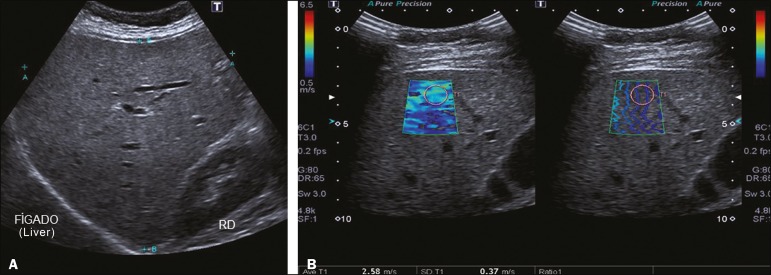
A: Ultrasound examination of a liver with mild steatosis and signs of chronic liver disease, as characterized by blunt edges, irregular contours, and heterogeneous parenchyma. B: A 2D-SWE study showing greater stiffness of the liver parenchyma (2.58 m/s), consistent with a Metavir stage of F4. On the elastography map, tones of green predominate, and there is greater line separation in the propagation mode.
